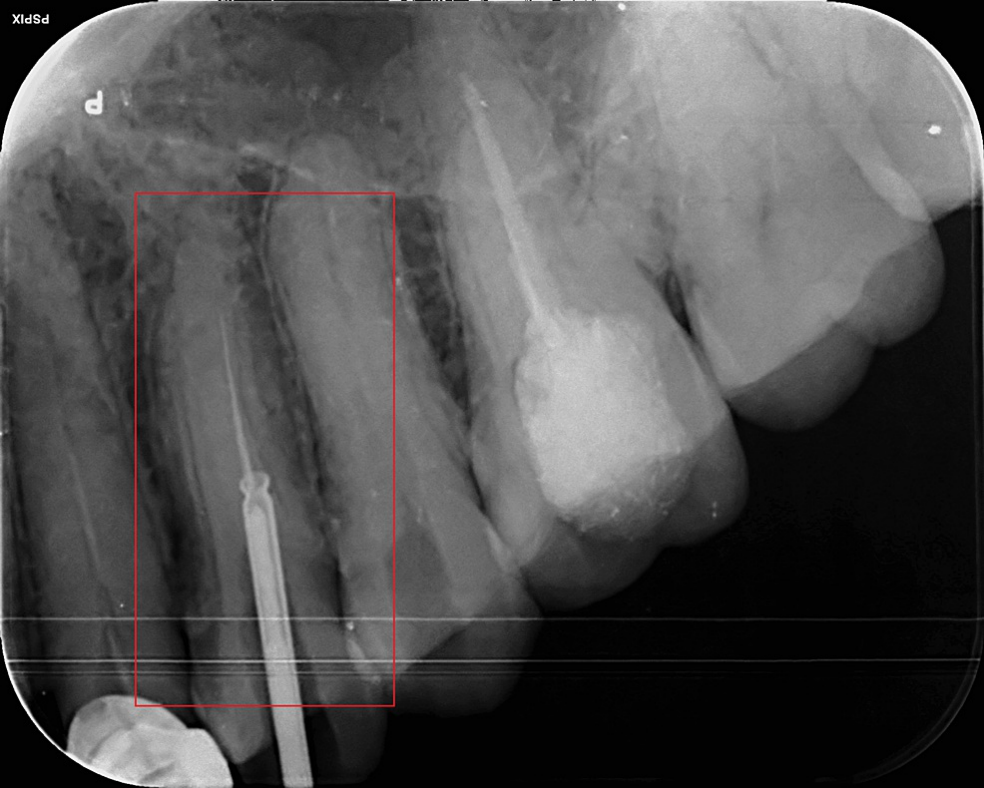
Radiographic confirmation of the location of the extractor in 24 The extractor was seen encircling 2 mm of the separated fragment in 24
